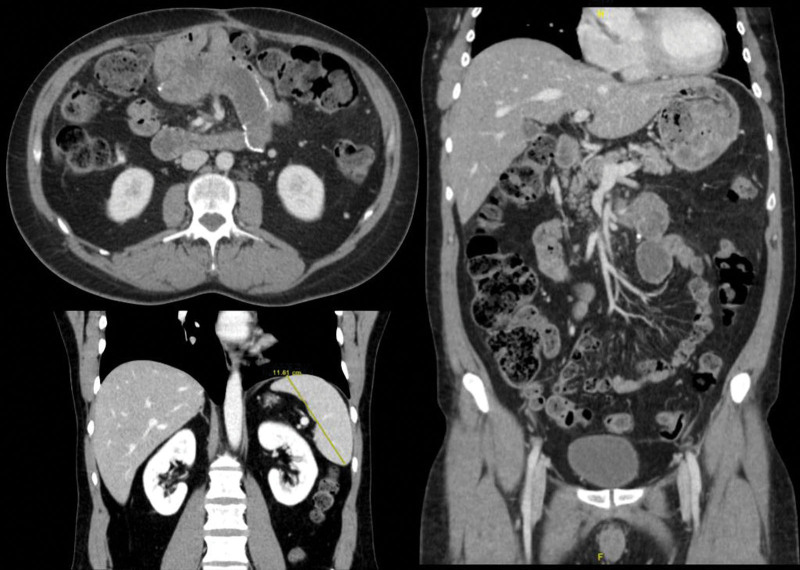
Images of the CT-scan performed in December 2021. It shows radiological improvement, without peritoneal abscesses nor mesenteric lymph node enlargement.
